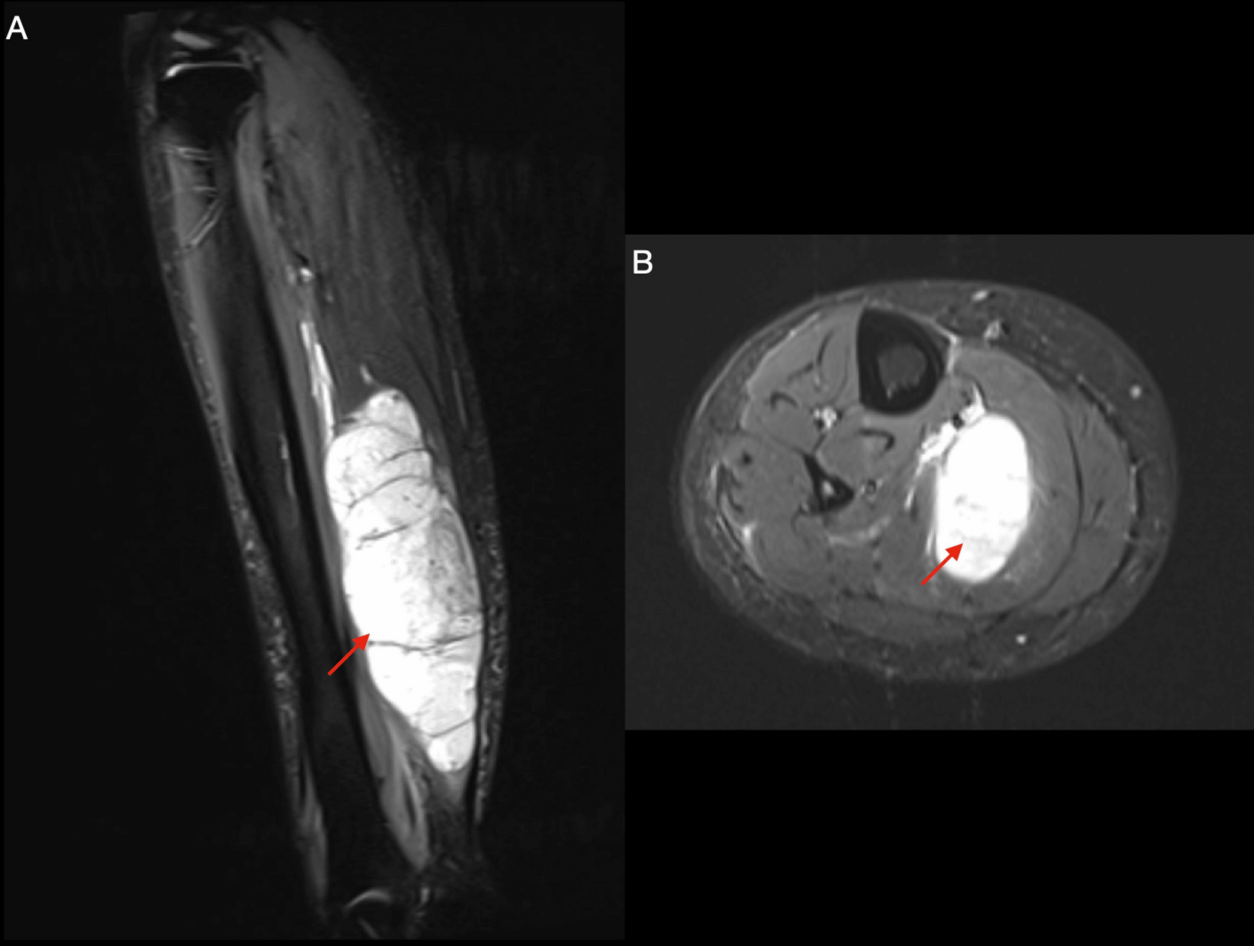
Sagittal (A) and axial (B) views of T2-weighted MRI of the right tibia and fibula, obtained 3.5 months after symptom onset, demonstrated a hyperintense intramuscular mass with internal septations (red arrows). Axial images showed intact gastrocnemius boundaries with a hyperintense lesion confined within the soleus muscle. The mass measures 182 x 51 x 54 mm in size.
